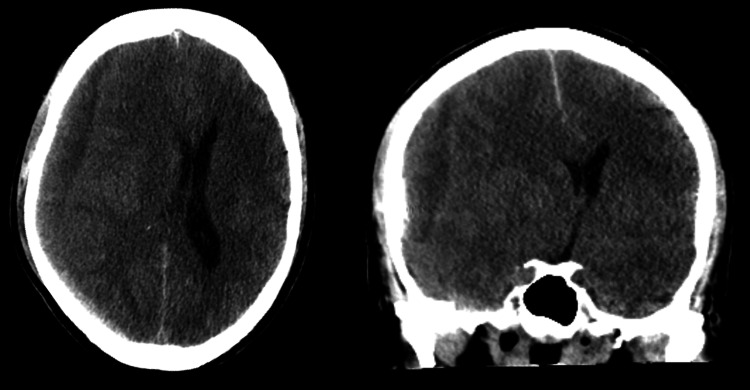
CT head axial (left) and coronal views (right) displaying intracranial hemorrhage expansion: 17 mm right subdural hematoma and 11 mm leftward midline shift with uncal herniation
